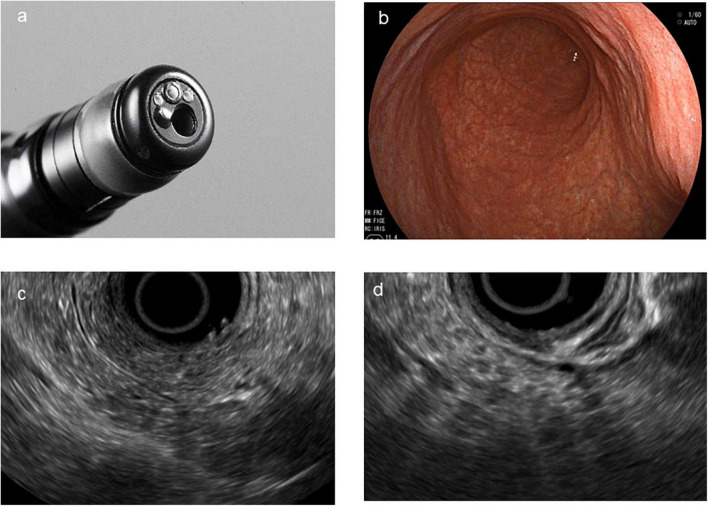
Direct forward-viewing radial endoscopic ultrasound (FR-EUS). (a) The image of the tip of FR-EUS. (b) Endoscopic view of the stomach. (c) EUS image showing echogenic foci, echogenic strands, and echogenic ductal walls. (d) EUS image showing typical lobularity.
